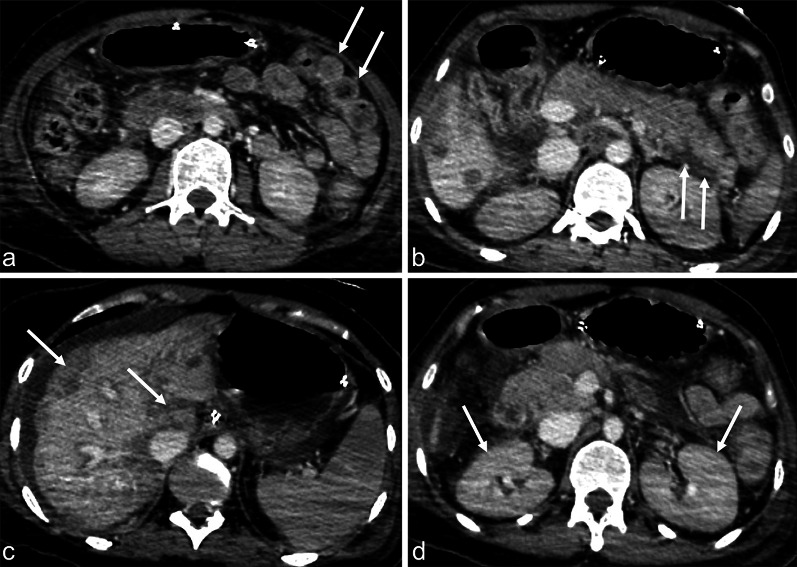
Contrast-enhanced CT images in the portal venous phase, in the axial view, showing a 46-year-old female with lymphoma and sepsis due to infection (qSOFA 2). A thickened and hyperdense bowel wall can be observed (a, arrows), with peripancreatic oedema (b, arrows), reduced and inhomogeneous liver enhancement (c), and enlarged kidneys with abnormal enhancement (d, arrows)
